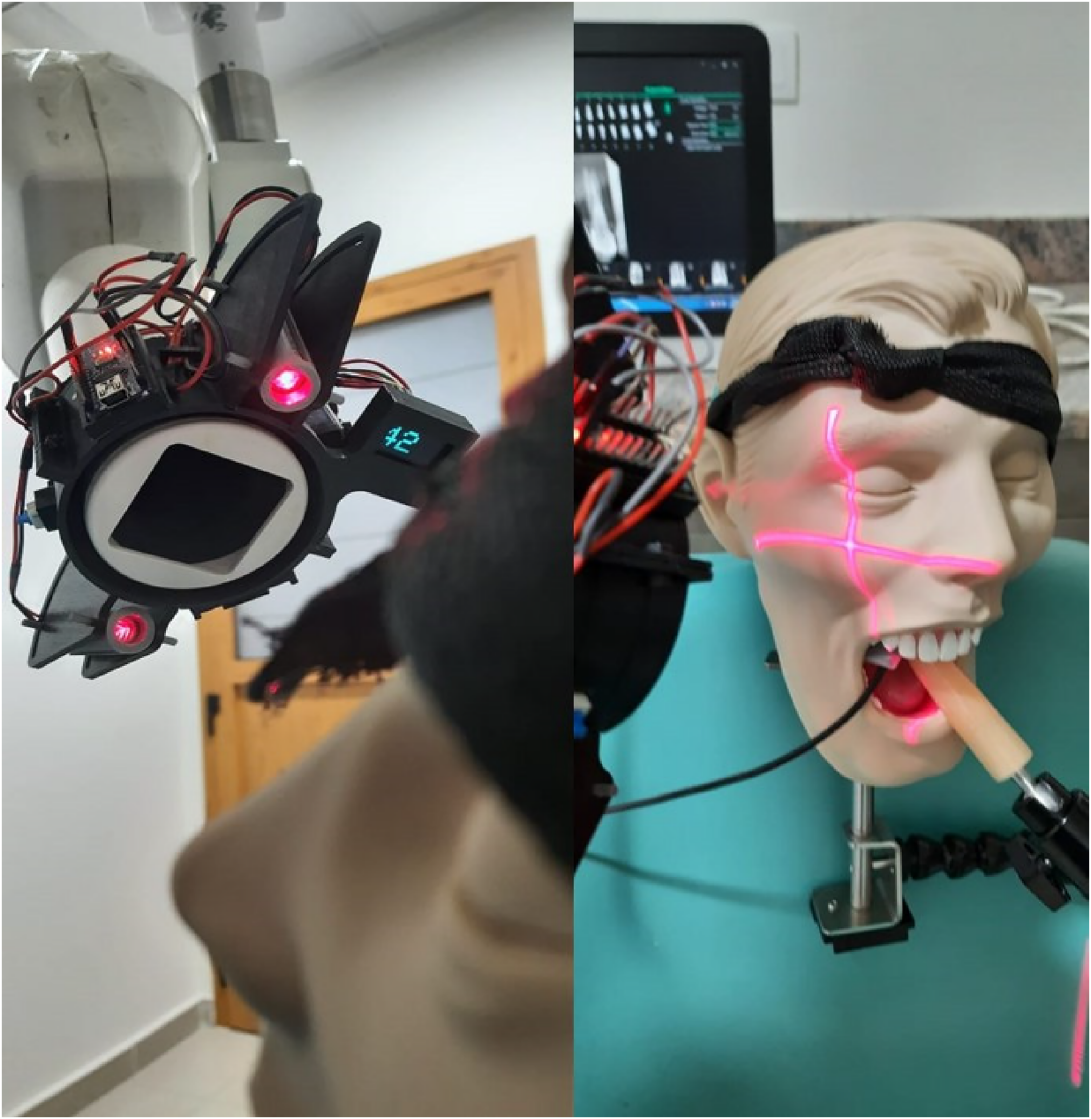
Shows Nallan's directRay LASER and gyroscopic device fitted on the x-ray machine for periapical projections.
